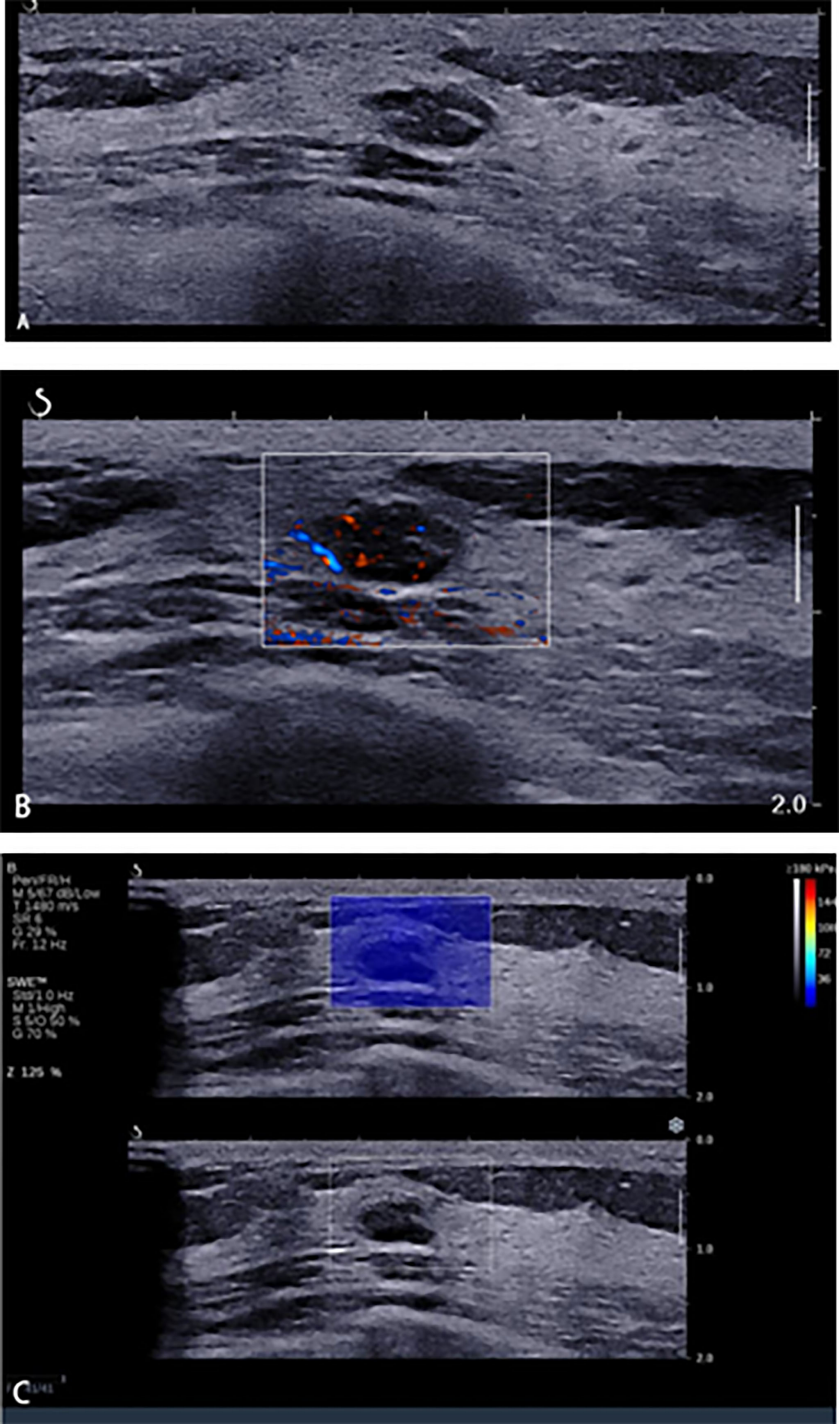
A 45-year-old woman with breast lesions. (A) Conventional B-mode ultrasound revealed a 10 × 6-mm round, hypoechoic lesion with a clear margin in the right breast, which was categorized as Breast Imaging Reporting and Data System (BI-RADS) 4a. (B) The morphologic and distribution features of the microvessels in AngioPLUS microvascular Doppler ultrasound technique (AP) followed a linear pattern. (C) The qualitative shear wave elastography (SWE) feature showed no finding. Considering the benign manifestation both in AP and qualitative SWE, the final category was downgraded into BI-RADS 3. The ultrasound-guided biopsy revealed the lesion as fibroadenoma.
